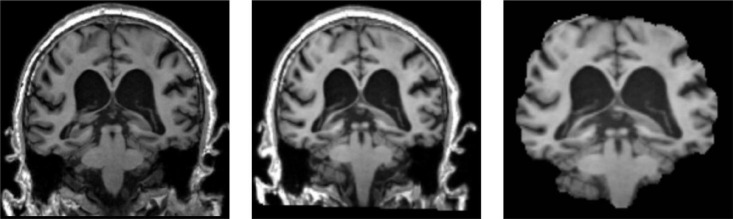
Sample slices of MRI images after registration and skull removal.
